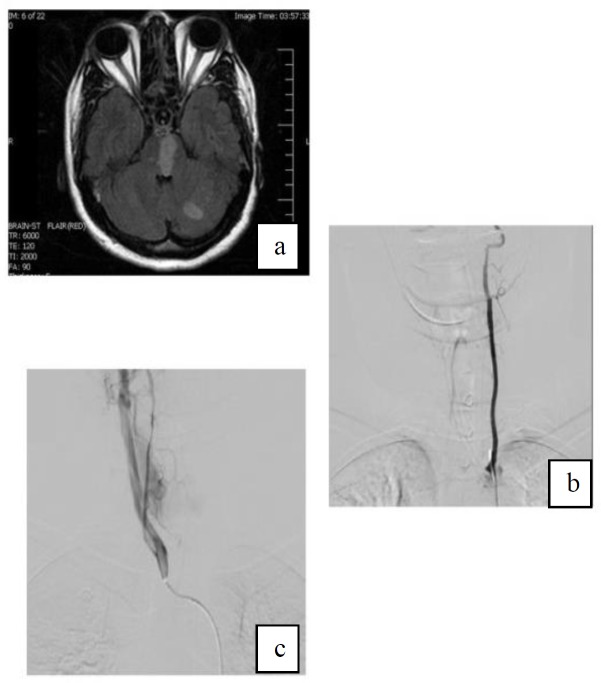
a) Axial T2-FLAIR weighted MRI depicts hypersignality in left cerebellum and medulla b) Cerebral DSA shows aberrant origin of left VA from aortic arch between left subclavian artery and left CCA c) Cerebral DSA realizes dissection in V2 and tapering at V4 due to occlusion of the lumen
